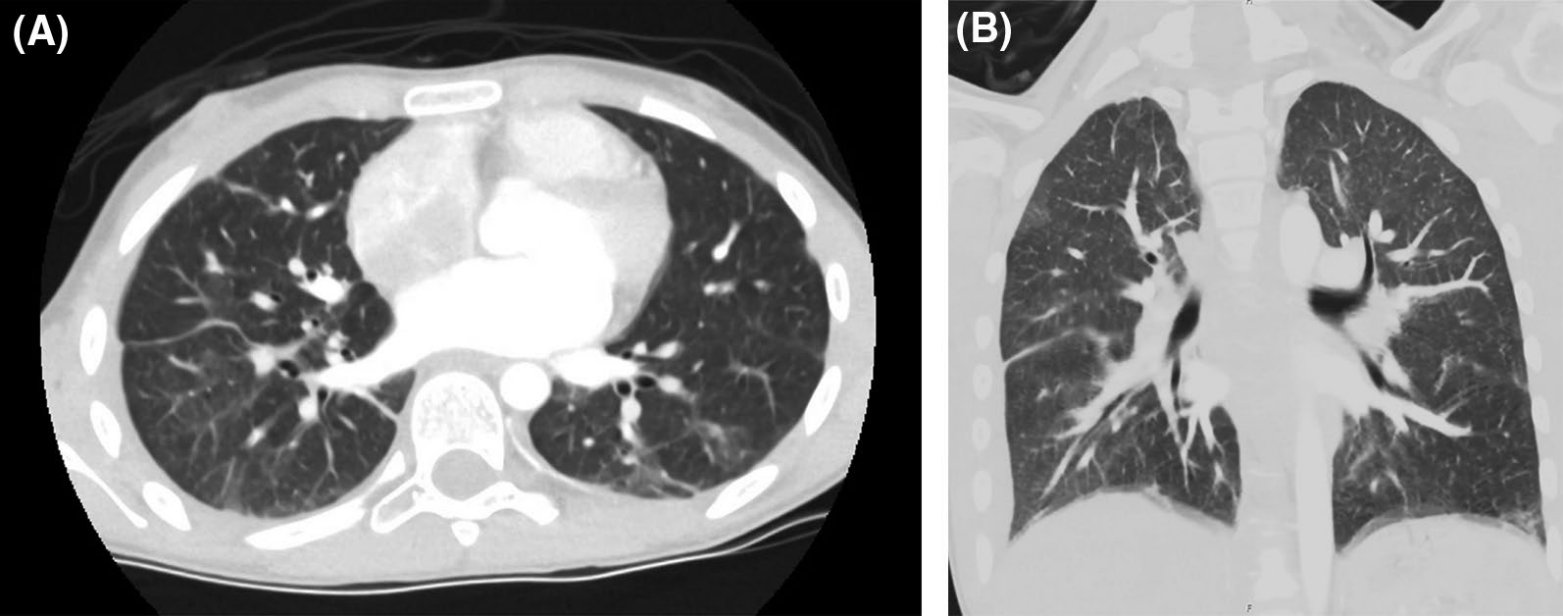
CT thorax obtained at 2-month follow up demonstrating near complete resolution of infiltrates. A Transverse plane; B coronal plane
